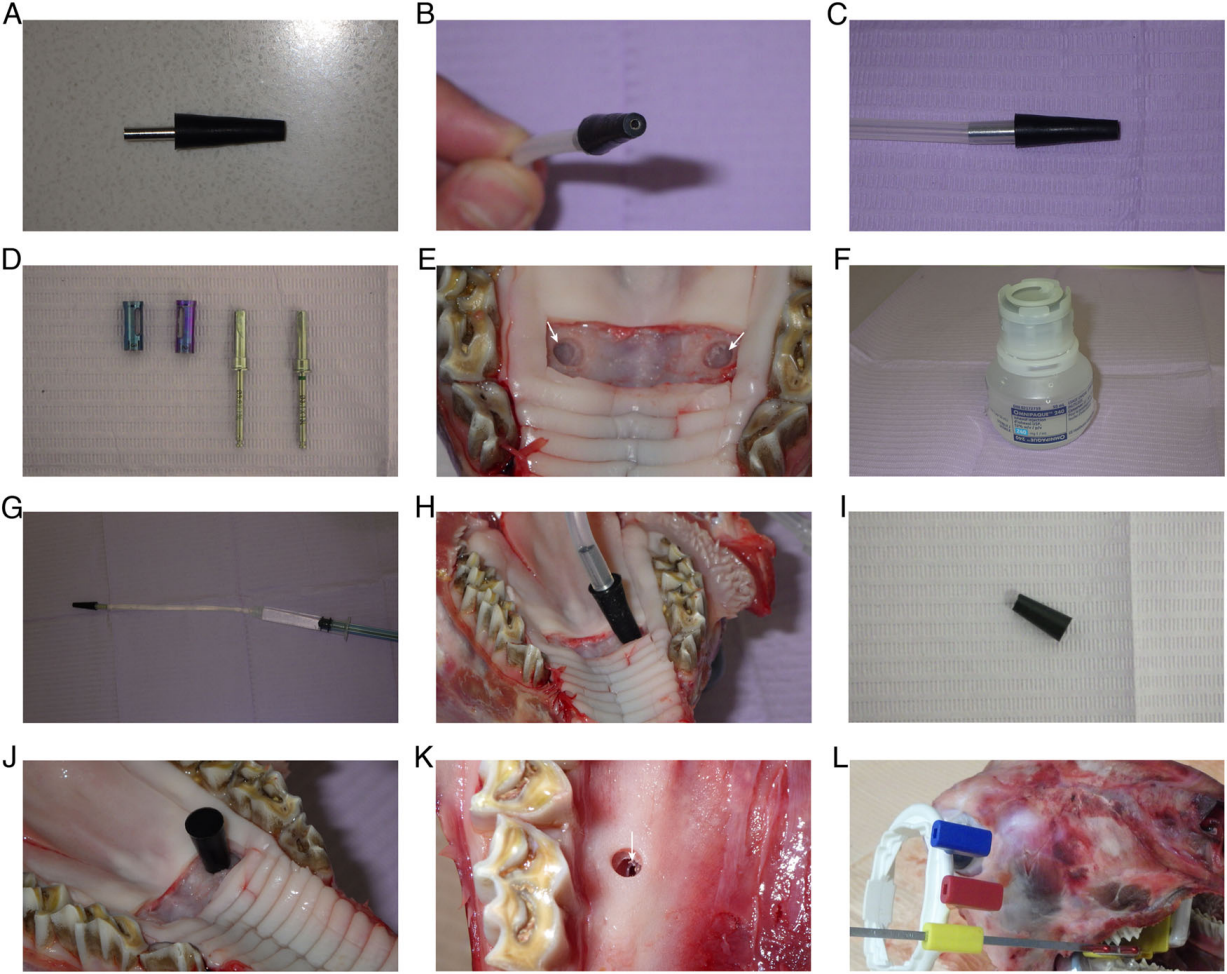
Clinical documentation of the intervention. A Specifically designed silicone retentive end combined with a metallic injection stent. B Top view showing how the metallic injection stent supports the silicone retentive end from within to prevent its deformation upon being inserted through the osteotomy. C Side view of the retentive end/ injection stent assembly combined with a silicone fluid supply tube. D The 3.1 mm and 3.8 mm diameter safe drills from CAS kit (Hiossen Canada) along with the 2 mm and 3 mm drill stoppers used to safely expose the sinus membrane. E Following the completion of both right and left side osteotomies, the white arrows show the intact exposed sinus membranes. F Omnipaque 240 contrast agent (GE Canada). G The specifically designed apparatus combined with a 3 ml graduated syringe loaded with contrast agent. H The self-retaining apparatus inserted into one of the osteotomies. I Silicone conical plug. J Self retaining conical plug inserted into the osteotomy following the fluid injection. K White arrow showing artificial perforation in the sinus membrane. L Extension cone paralleling device in place prior to periapical radiograph acquisition.
